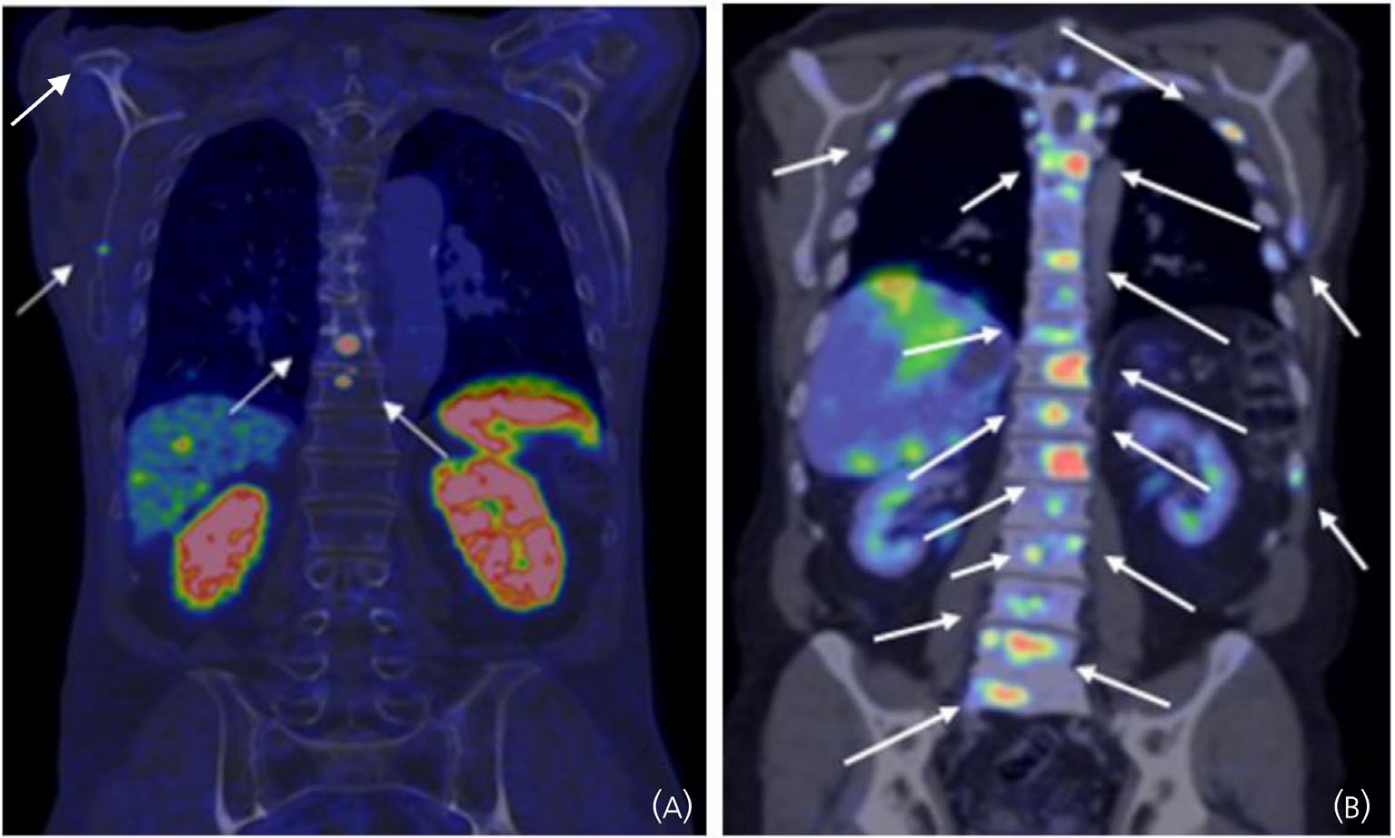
(A) ^68^Ga‐DOTATOC PET/CT (coronal PET–CT fusion) of an 83‐year‐old male with Si‐NET and ≤ 5 bone metastases indicated with arrows. (B) ^68^Ga‐DOTATOC PET/CT (coronal PET–CT fusion) of a 62‐year‐old male with Si‐NET, liver metastases and multiple bone metastases (>5) indicated with arrows. CT, computed tomography; ^68^Ga‐DOTATOC‐PET/CT, Gallium‐68‐labelled DOTA tyrosine octreotide positron emission tomography with computed tomography; PET, positron emission tomography; Si‐NET, small intestinal neuroendocrine tumour.
